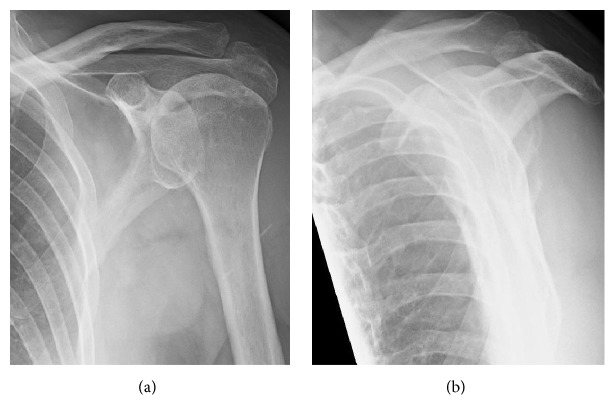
Anterior-posterior (a) and lateral (b) radiographs of our patient's shoulder prior to the arthroscopy (and prior to the dislocations).
